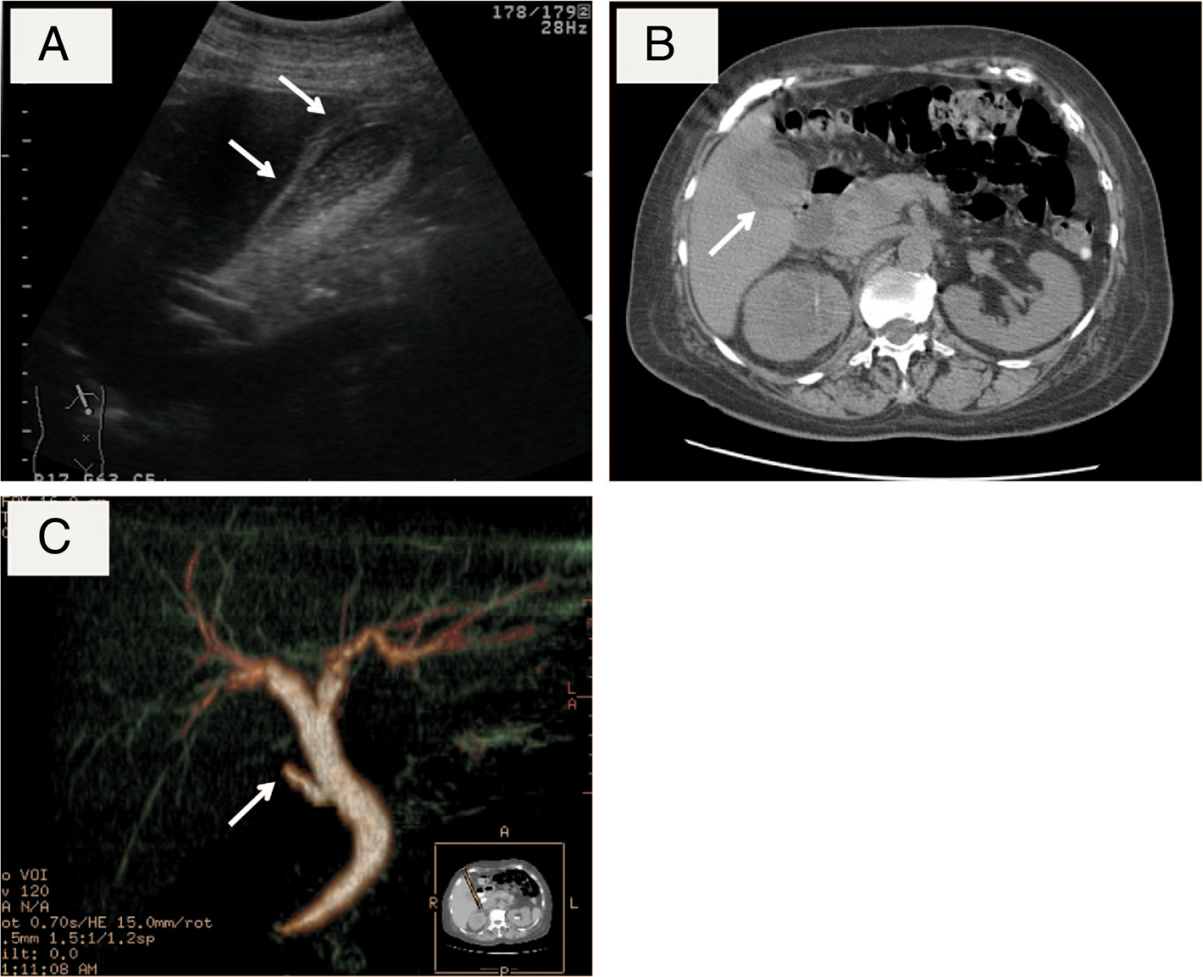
a US showed thickening of the gallbladder wall and subserosal edema. b CT showed the enlarged gallbladder and the thickened gallbladder wall. c DIC-CT showed no gallstones in the common bile duct and an interruption of cystic duct
